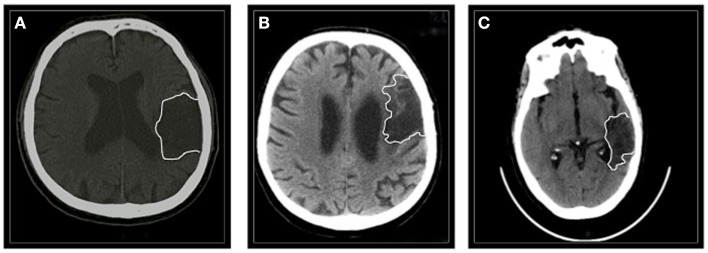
The figure shows a single CT scan acquisition of all the three patients. (A) Patient #1 suffered from a left (fronto)temporal hemorrhage. (B) Patient #2 had a left frontal ischemic stroke. (C) Patient #3 had a left temporal hemorrhage.
